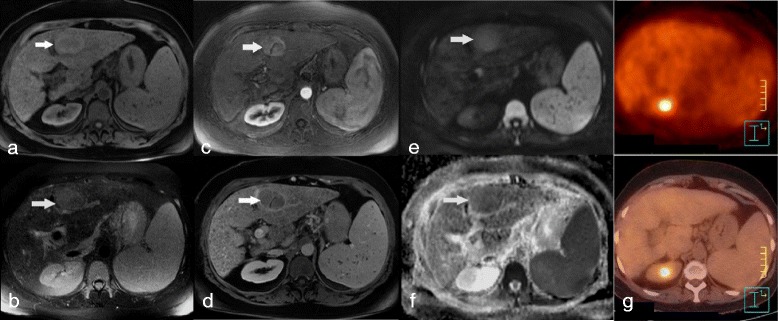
Moderately differentiated (grade 2) HCC in a patient with hepatitis B-induced cirrhosis, showing restricted ADC on DWI-MRI and no significant uptake on FDG-PET/CT. MR imaging showed a left lobe HCC with high T1 (a, *arrow*), high T2 (b, *arrow*) signal intensity, with arterial enhancement (c, *arrow*) followed by washout (d, *arrow*). The HCC showed high signal intensity on DWI image (b = 800 s/mm^2^; e, *arrow*) consistent with low ADC (1.26 × 10^−3^ mm^2^/s) on the ADC map (f, *arrow*). The lesion showed no significant FDG uptake on FDG-PET/CT (g)
